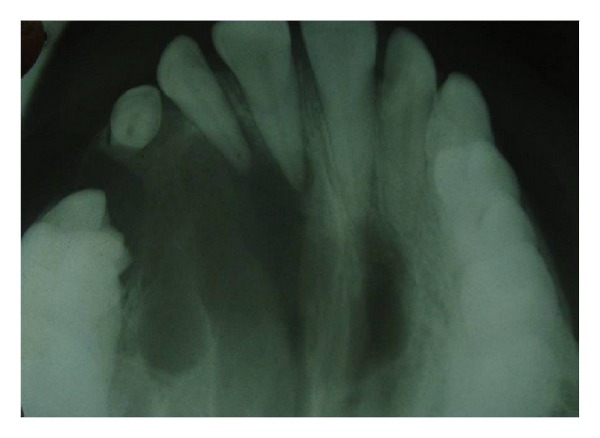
Maxillary cross-sectional occlusal radiograph showing radiolucency involving the right maxilla.
